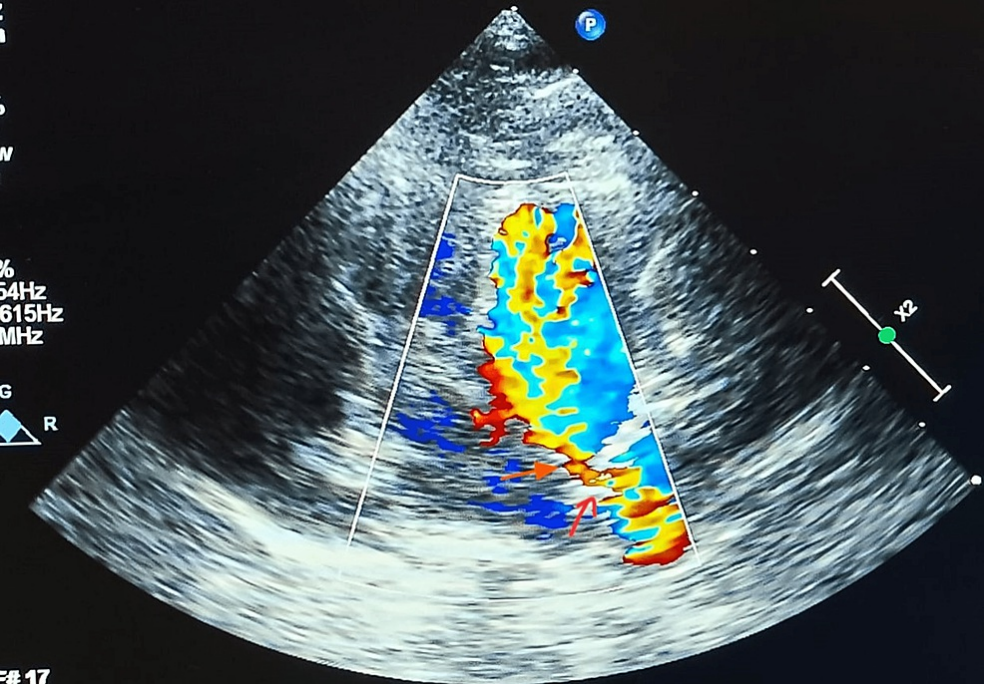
2D Echo: Parasternal Short Axis View Showing PDA PDA: Patent ductus arteriosus The image has been taken by the authors.
